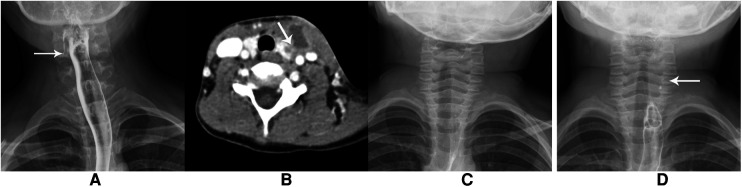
(A) A 10-year-old girl had a recurrent infection in the right neck for 8 years. The barium esophagography showed the strip high-density shadow on the right side, indicating pyriform fossa fistula. (B–D) A boy aged 4 years and 10 months old had a recurrent mass in the left neck for 3 years. (B) contrast-enhanced CT showed the formation of an abscess in front of the left neck and involvement of the left lobe of the thyroid. (C) The barium esophagography showed normality 3 days after infection. (D) The esophagography showed punctate contrast agent residue in the left neck one month later, suggesting pyriform fossa fistula.
